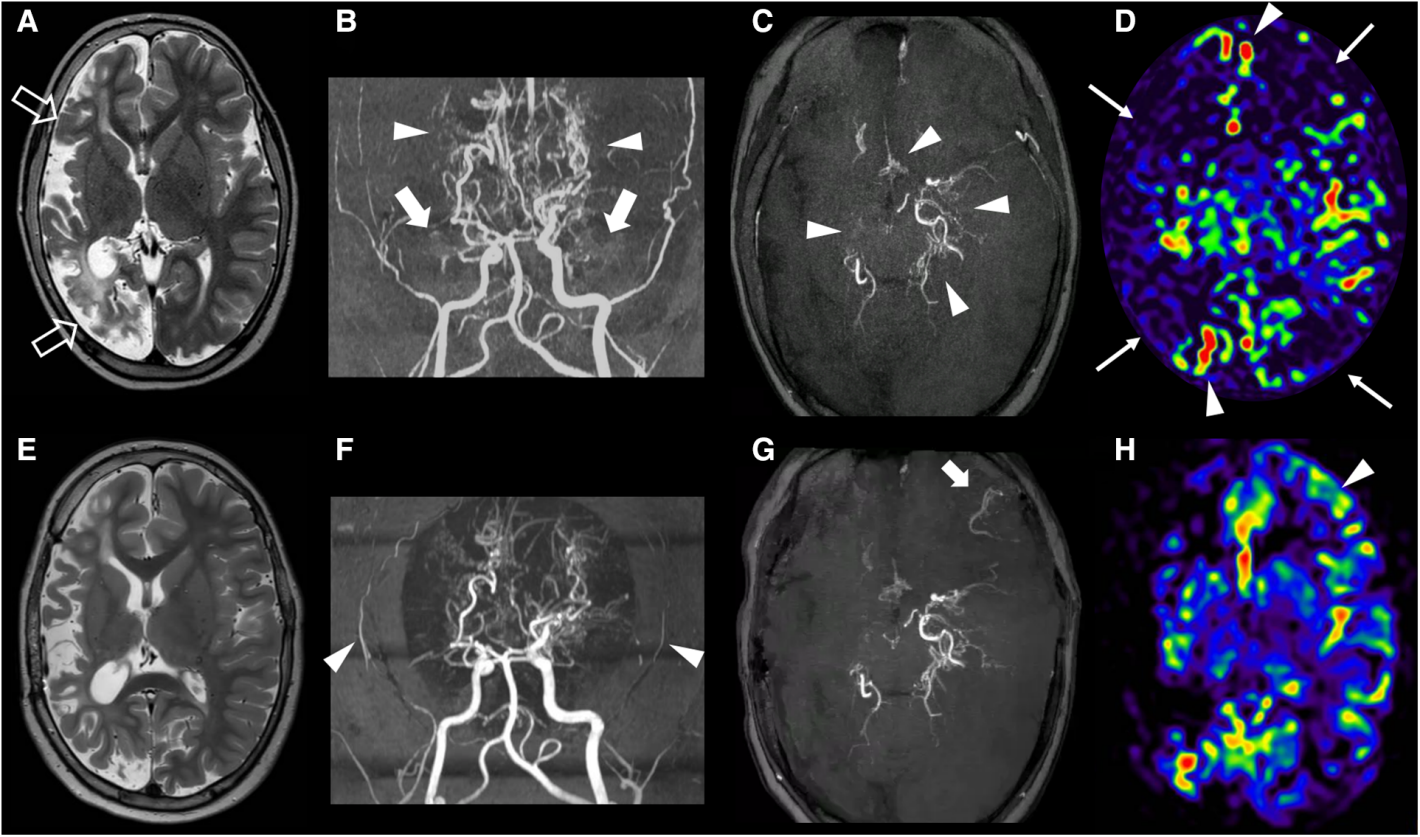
Clinical and imaging findings. Brain MRI and MR angiography of the subject performed before revascularization at 8 years of age (A–D) and at last follow-up, after eleven months (E–H). A) Axial T2-weighted image shows a chronic arterial ischemic infarct in the right cerebral hemisphere (empty arrows) with relative sparing of the mesial frontal and insular regions. (B,C) Brain MR angiography with 3D reconstruction, frontal view (B) and axial MinIP reconstruction (C) demonstrate bilateral stenosis of the right supraclinoid portions of the internal carotid arteries with absent flow signal in the middle cerebral arteries (thick arrows) and anterior cerebral arteries, and multiple moyamoya collaterals (arrowheads). Note the initial distal stenosis of the left posterior cerebral artery. (D) Brain arterial spin labeling MR perfusion reveals reduced signal in the right cerebral hemisphere and in the left anterior frontal and parietal lobes (thin arrows) with bilateral arterial transit artifacts (arrowheads). (E) Follow-up axial T2-weighted image performed 11 months after the revascularization procedure shows no other ischemic infarcts. (F,G) Corresponding MR angiography images reveal reduction of flow signal in the superficial temporal arteries (arrowheads), absence of collateral formation at surgical site on the right, and a limited region of revascularization in the left fronto-basal region (thick arrow). (H) Arterial spin labeling MR perfusion demonstrated increased CBF only in the left fronto-basal region (arrowhead).
